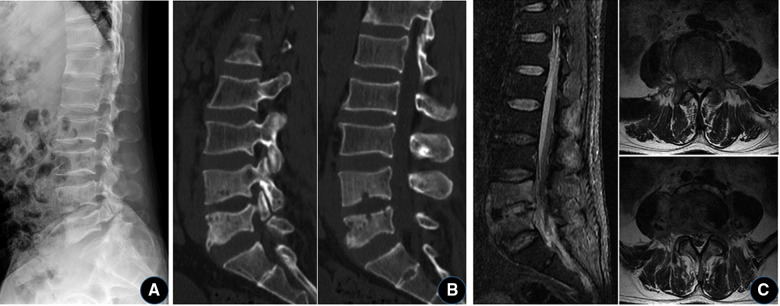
A 40-year-old man with Brucella lumbar infection and bilateral isthmus of the L5 vertebral body. (A) Plain x-ray shows degeneration of the lumbar spine, with a slight narrowing of the L4/5 intervertebral space and bilateral isthmus rupture at L5. (B) CT showed “worm-eaten-like” bone destruction in the L4 and L5 vertebral bodies, and L5 lumbar spondylolysis. (C) MRI showed abnormal signal in the L4/5 vertebral body and space, and the formation of abscess in the spinal canal.
